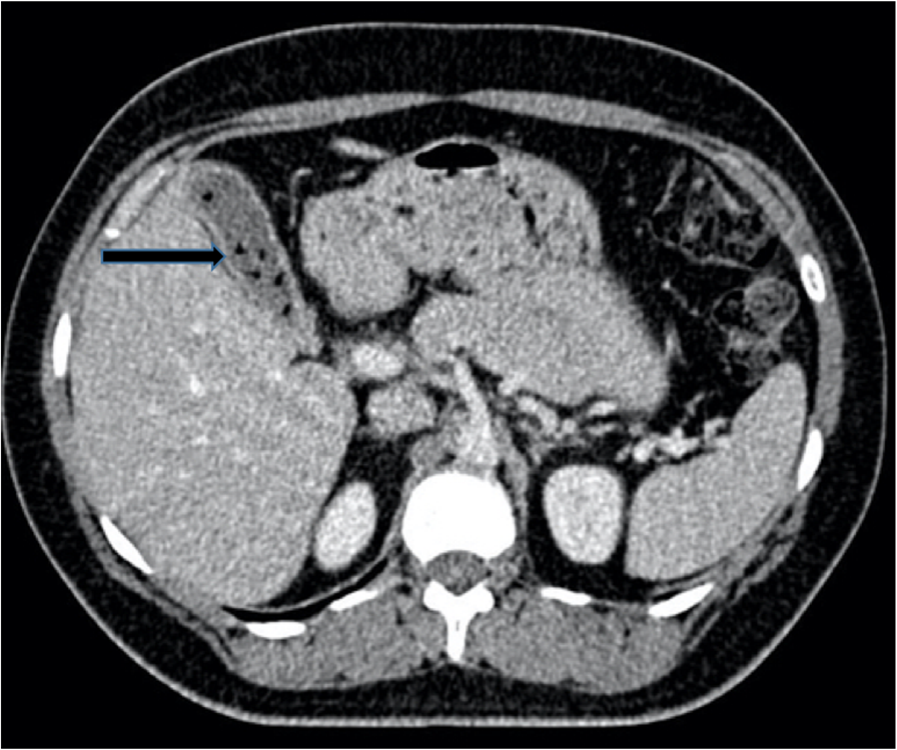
Axial contrast-enhanced CT of the abdomen. There are multiple small gallstones within the gallbladder which are hypoattenuating relative to the surrounding bile
